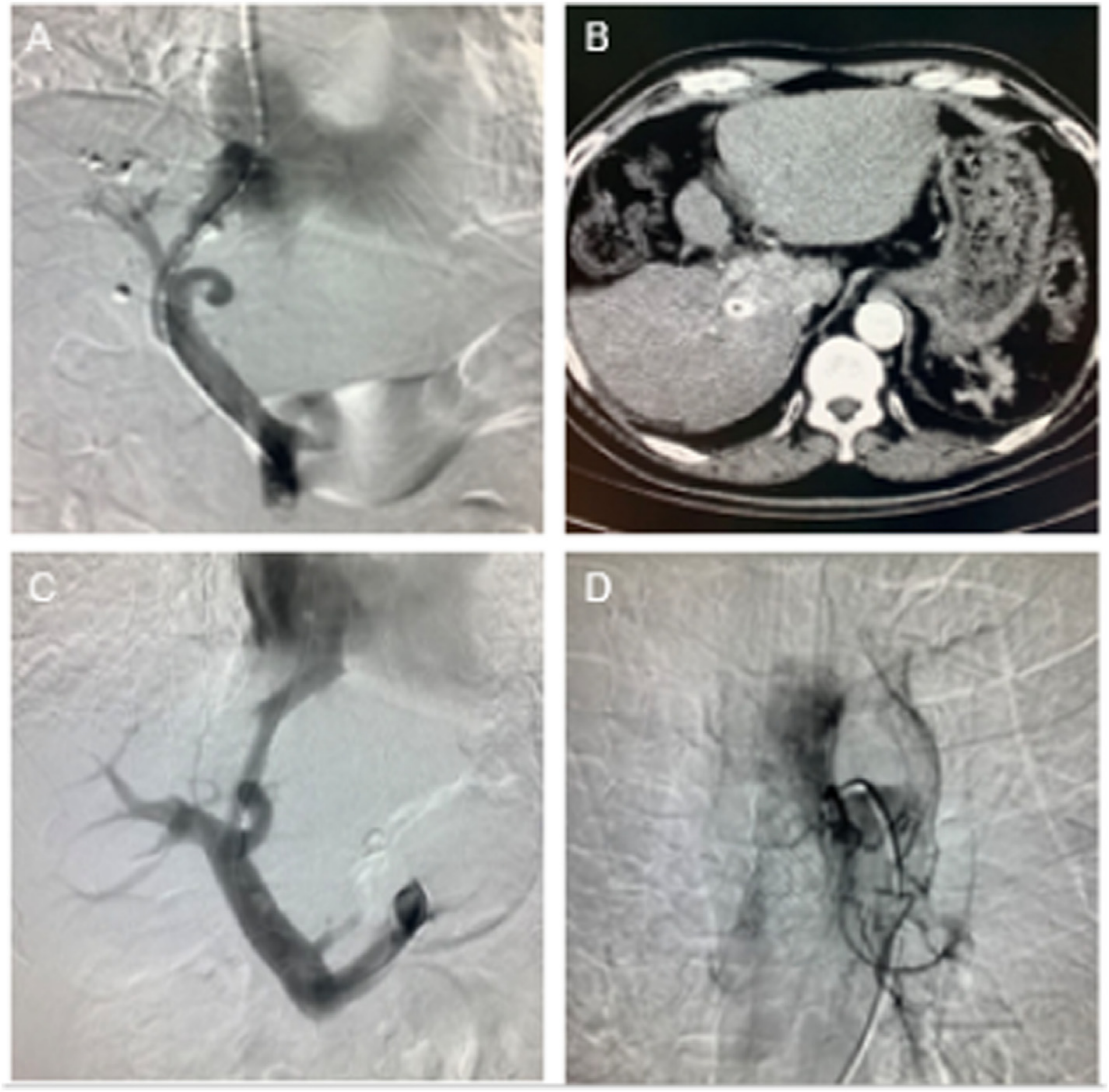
Picture A and B: Digital subtraction angiography (DSA) and postoperative computed tomography (CT) images of the patient with liver cancer combined with portal hypertension. Picture C and D: The TIPS and esophageal arterial chemoembolization DSA images of the patient with esophageal cancer and portal hypertension.
